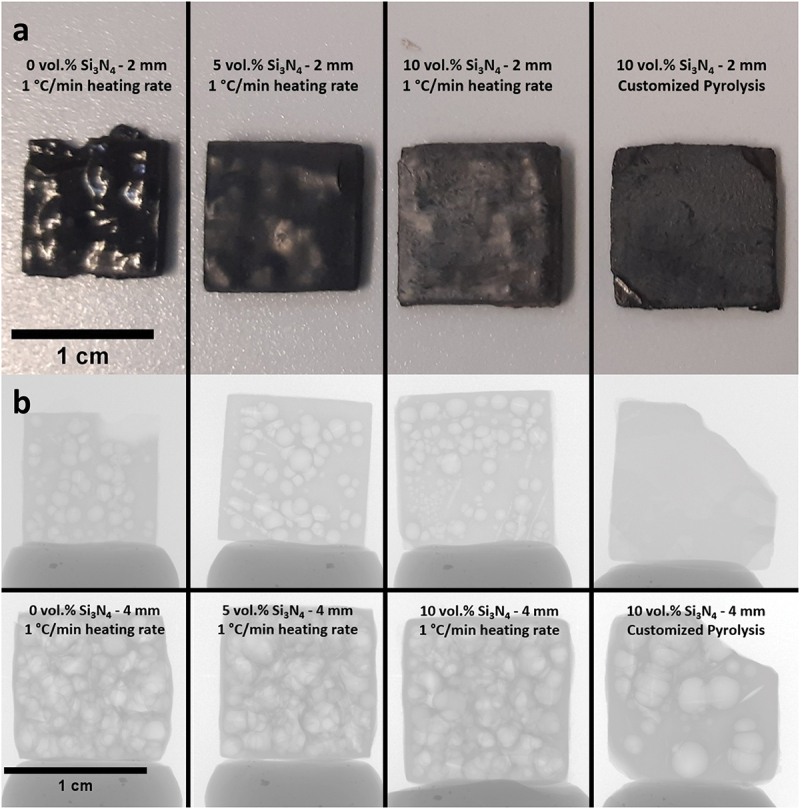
(a) 2 mm SiCN square prisms with 0, 5 and 10 vol.% Si_3_N_4_ loadings pyrolyzed at 1°C/min heating rate and the 2 mm 10 vol.% Si_3_N_4_-loaded square prism with customized pyrolysis profile; (b) the radiography images of pyrolyzed (1°C/min heating rate) SiCN samples with different Si_3_N_4_ loadings (0, 5, 10 vol.%) and thicknesses (2, 4 mm) as well as the 10 vol.% Si_3_N_4_-loaded samples with customized heating profile (2, 4 mm).
